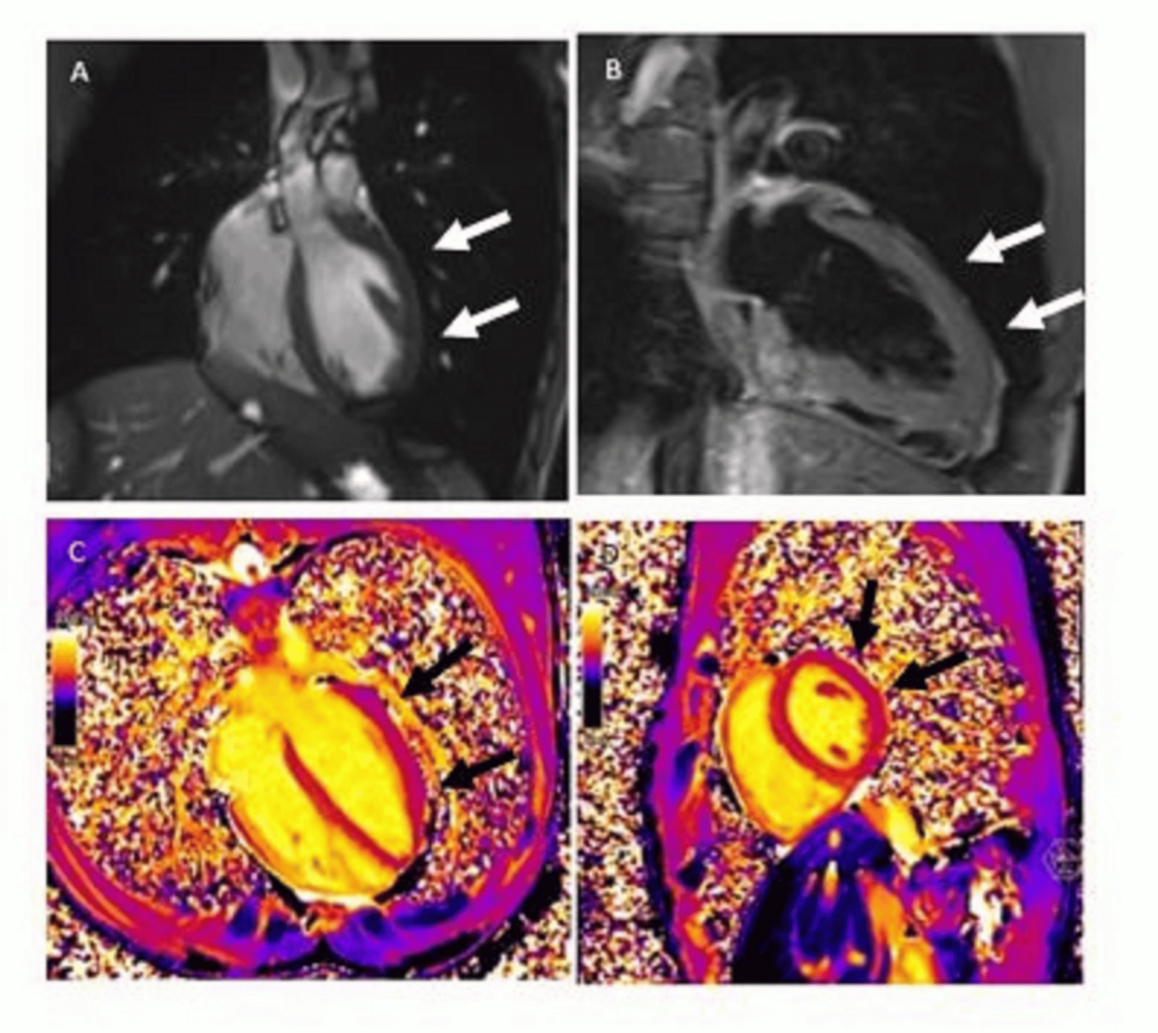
Cardiac magnetic resonance T2-weighted (A, B) and T2-mapping (C, D) images showing myocardial edema in the infero-septal mid-segment.
